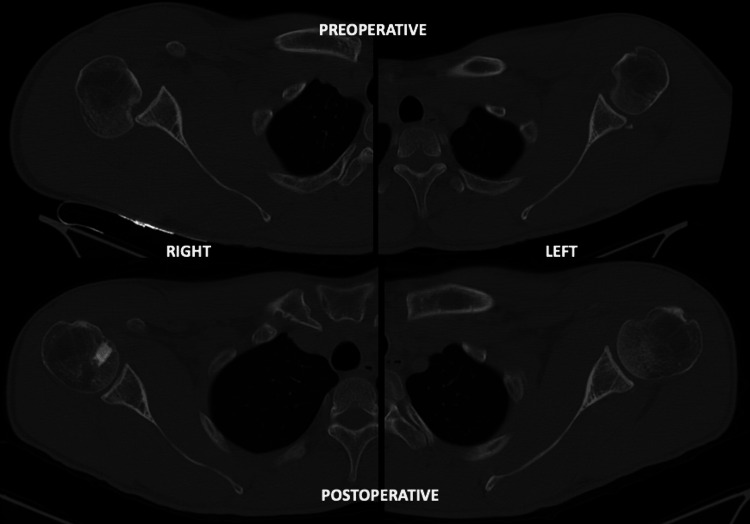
Axial computed tomography comparing preoperative and postoperative RHSL reduction. The image displays axial scans comparing the preoperative and postoperative views of a Reverse Hill-Sachs Lesion (RHSL). The preoperative scan shows the extent of the defect, while the postoperative scan demonstrates a nearly anatomic reduction of the lesion, indicating successful restoration of the humeral head contour and joint congruency.
